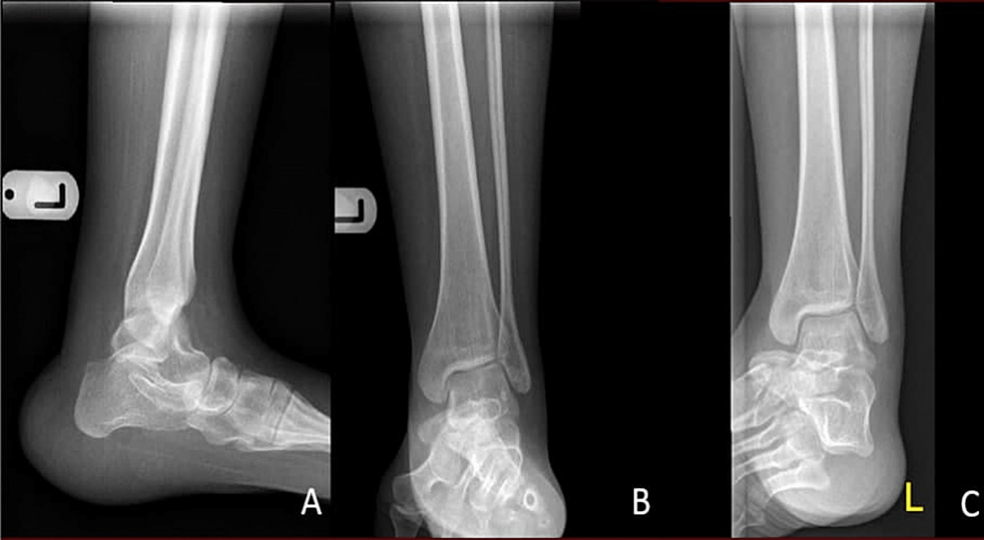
Preoperative X-ray of the left ankle: (A) lateral, (B) anteroposterior, and (C) mortise views
